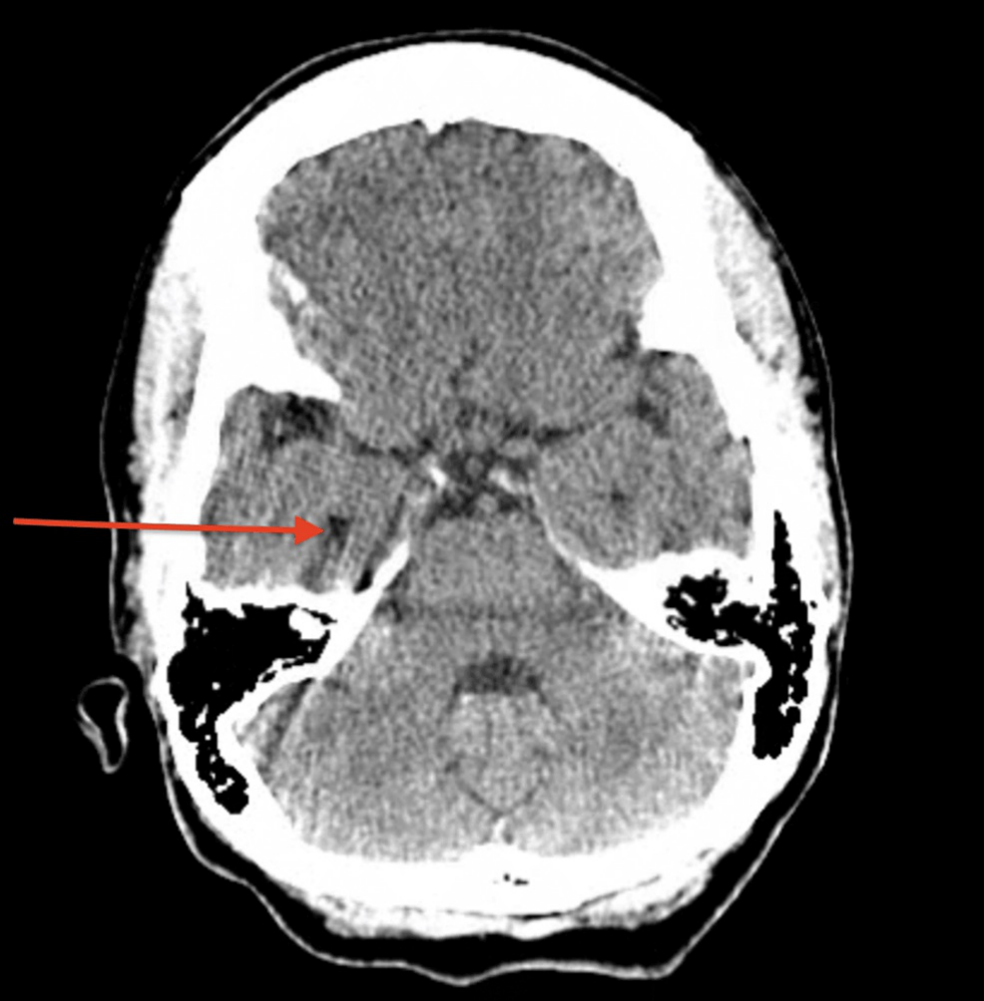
CT scan (coronal view) of the head without contrast The image is showing no acute abnormality (as demonstrated by the red arrow).
